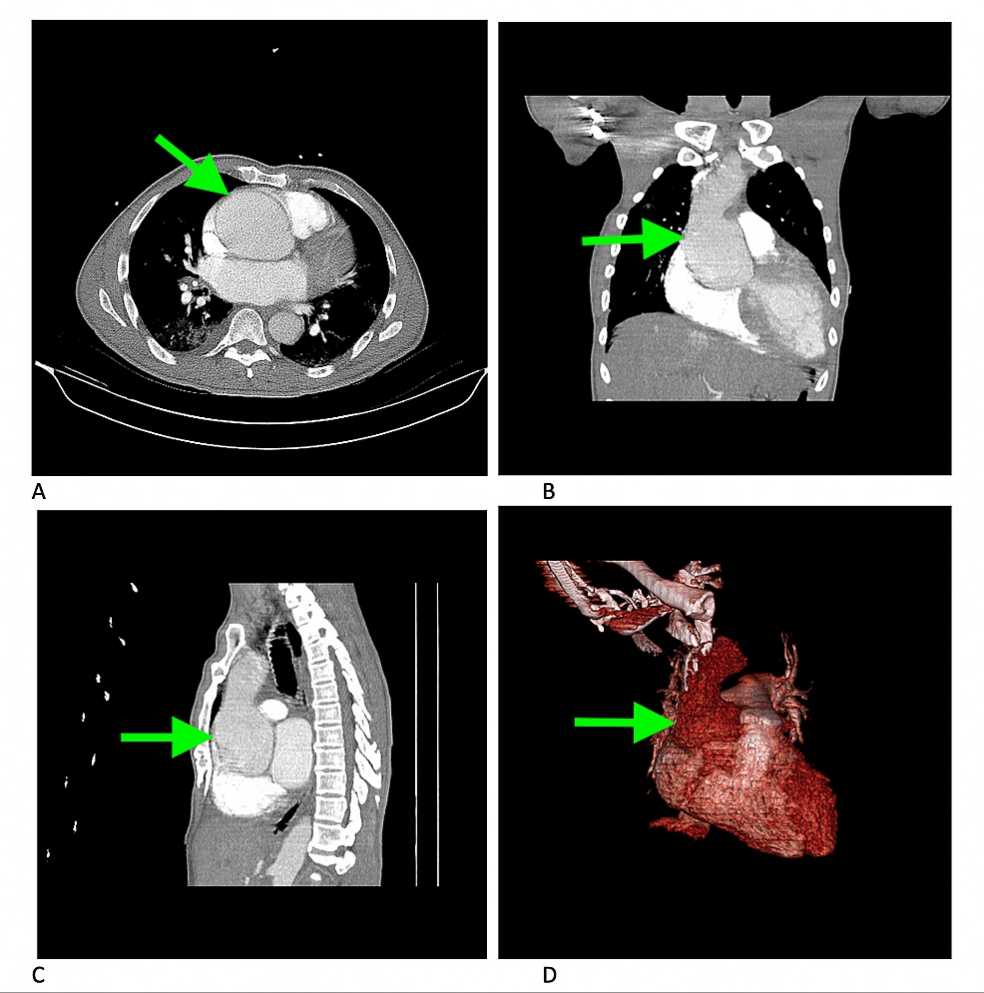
Chest CT angiography CT angiography of the chest demonstrating ascending aortic aneurysm (arrows) in axial (A), coronal (B), sagittal (C), and 3D reconstruction view (D)
